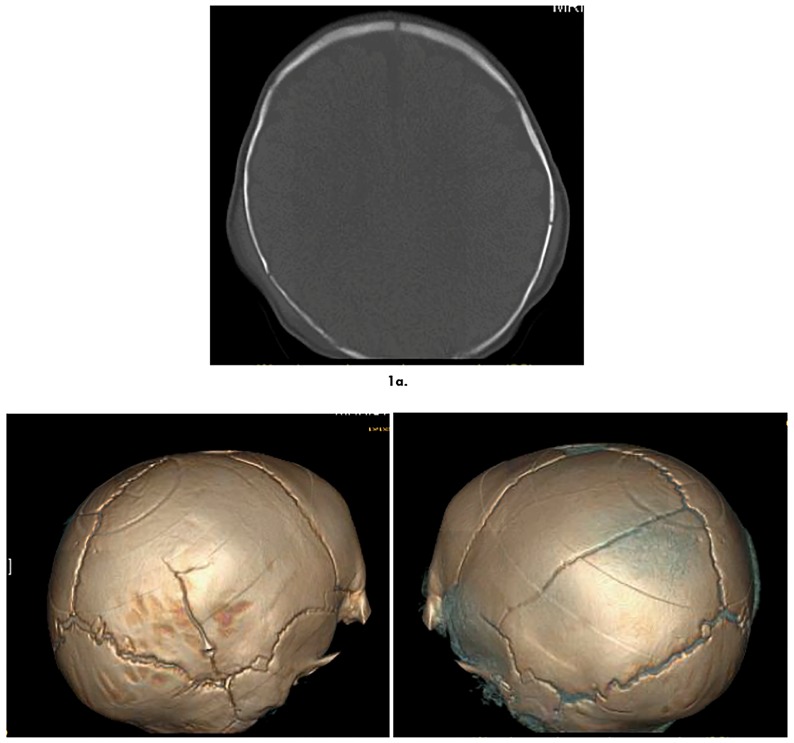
1a. Head CT coronal view demonstrating bilateral skull fracture with overlying soft tissue edema. 1b. 3D reconstruction images show asymmetric biparietal skull fractures.
